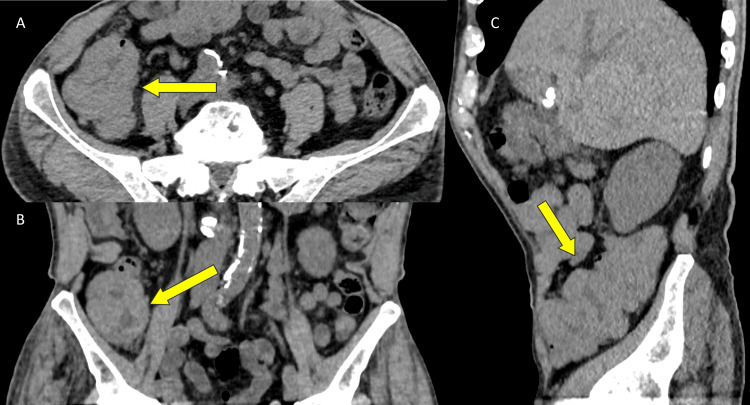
A. Axial, B. Coronal, and C. Sagittal images from a non-contrast CT exam demonstrating mural wall thickening of the ascending colon (yellow arrows)
